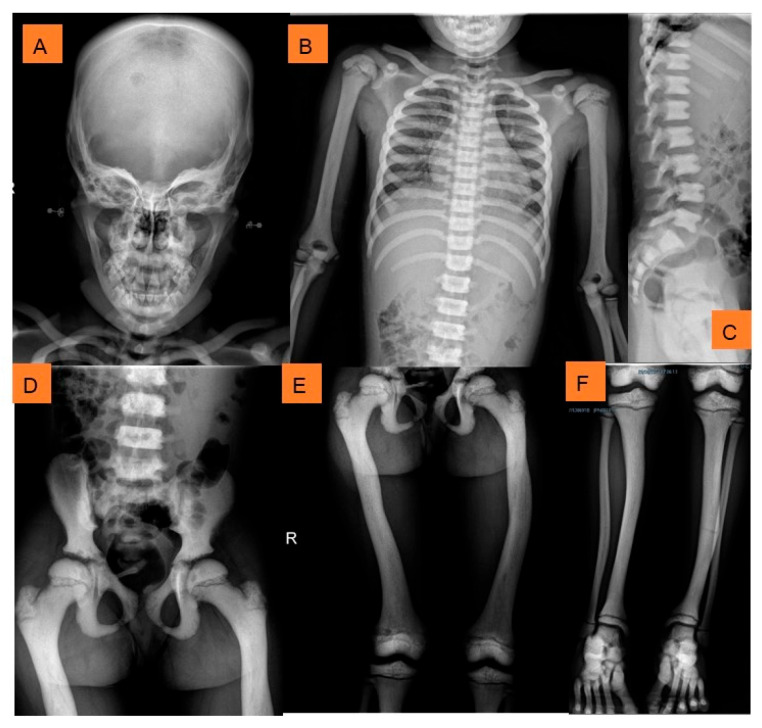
Radiographic features consistent with pycnodysostosis. (A) Posteroanterior skull view showing increased bone density and calvarial thickening. (B) Thoracic X-ray showing hypoplasia of the lateral (acromial) ends of the clavicles. (C) Lateral spine radiograph showing spool-shaped vertebral bodies with anterior fishtail deformities. (D,E) Radiographs of the pelvis and femurs showing diffuse osteosclerosis and a healed fracture with deformity of the left femur. (F) Tibia and fibula radiographs showing diffuse sclerosis, bowing, and a transverse midshaft fracture of the left tibia.
